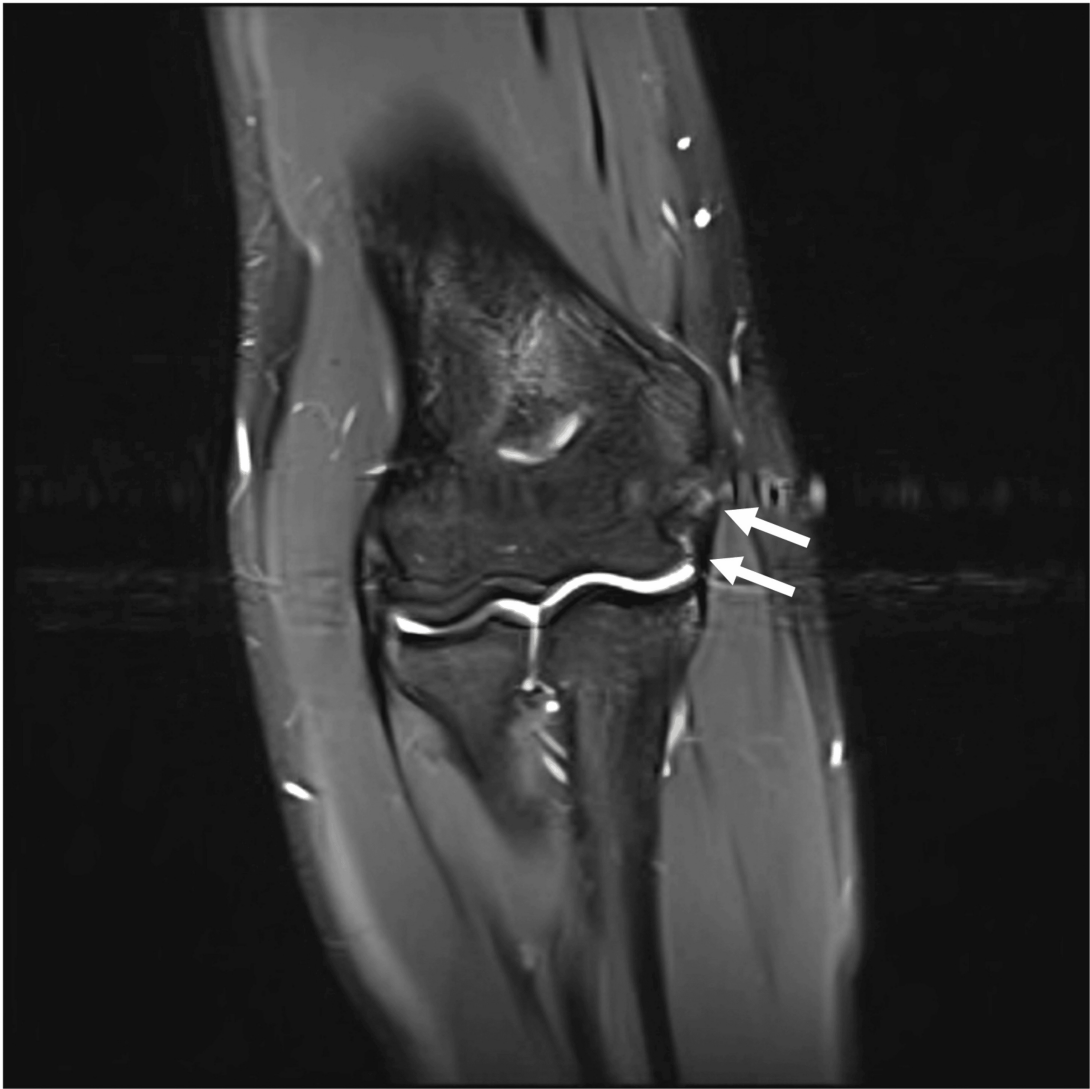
Coronal STIR MRI view of a 17-year-old man showing UCL partial tear and mild osseous irregularity at the attachment of the UCL, which is mildly thickened anteriorly with minimal edema. Short T2 inversion recovery (STIR); magnetic resonance imaging (MRI); arrows: ulnar collateral ligament (UCL).
